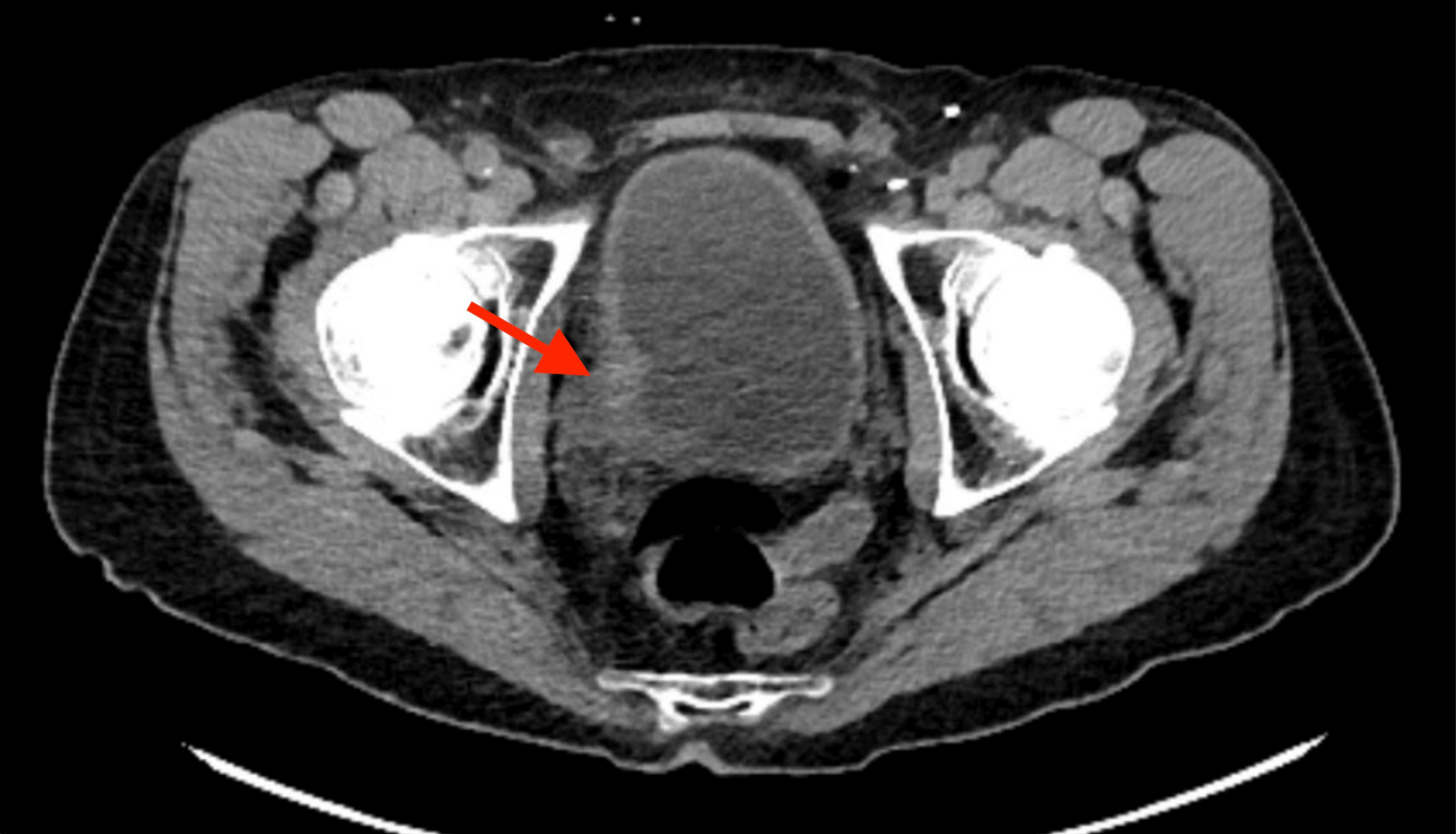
CT pelvis depicting enhancing mass within the right posterolateral wall of the bladder CT: computed tomography
